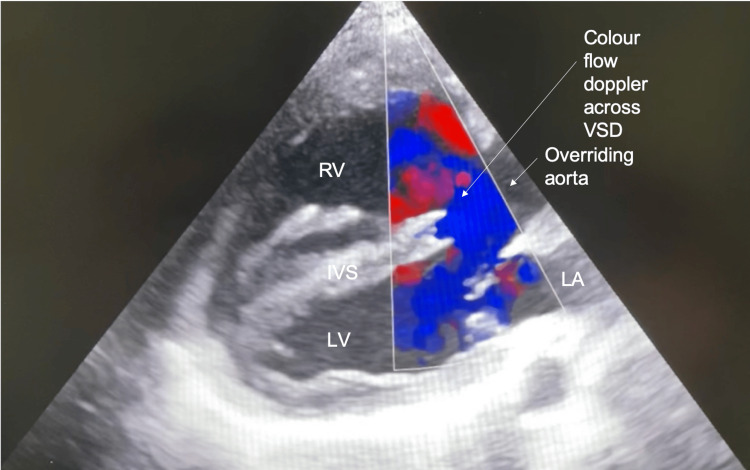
Parasternal long-axis view with colour flow Doppler across a perimembranous ventricular septal defect LA: Left atrium; LV: left ventricle; RV: right ventricle; IVS: intraventricular septum; VSD: ventricular septal defect
